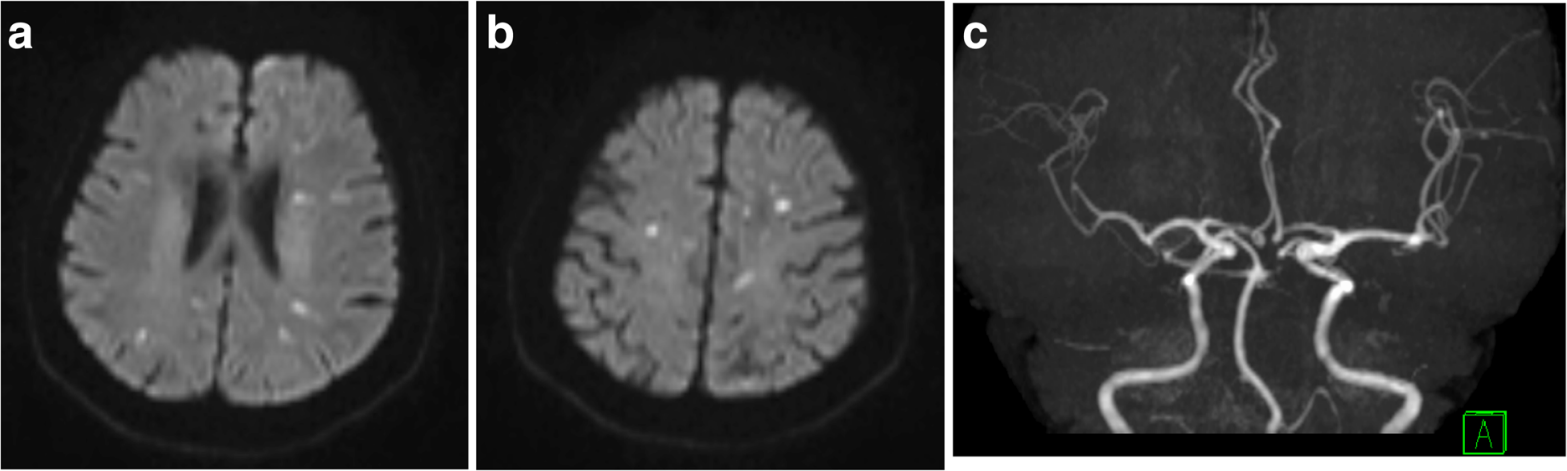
Brain magnetic resonance imaging (MRI). Axial diffusion-weighted brain MRI showing multiple hyperintense spots in the bilateral cerebral subcortical white matter (a, b). No intracranial arterial occlusion or stenosis was detected by magnetic resonance angiography (c)
